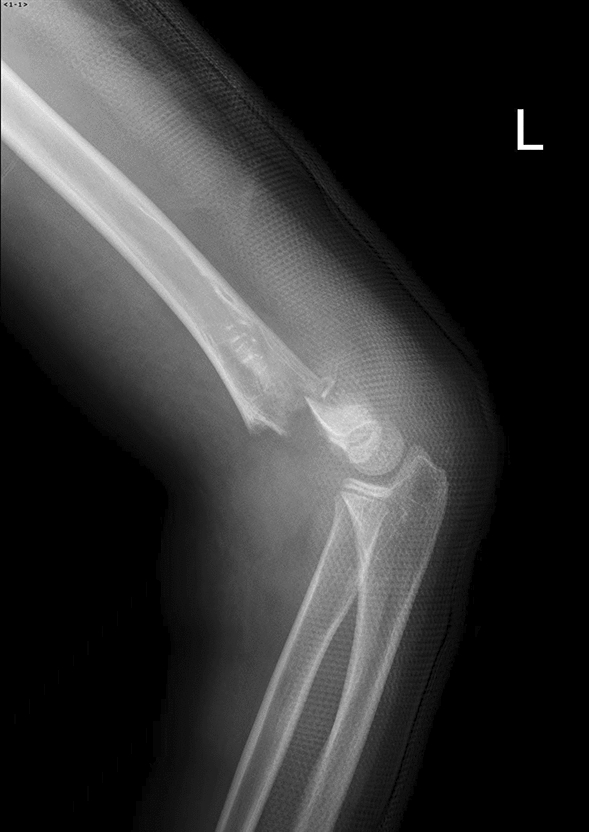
Post-injury X-ray in the lateral view shows a fracture at the humeral condyle, with complete backward displacement of the distal bone fragment
